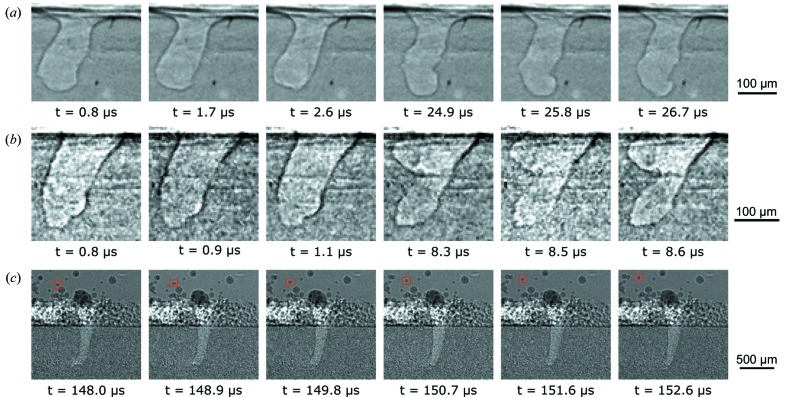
High-speed X-ray images of (*a*) and (*b*) the laser AM process for the Al–Si10–Mg plate, and (*c*) the powder-bed fusion process for Ti–6Al–4V. The frames are cropped such that the details of the vapor depression can be clearly identified as *e.g.* oscillations in the liquid–vapor surface. The experimental parameters were: (*a*) laser power 520 W, scan speed 0.8 m s^−1^, frame rate 1.08 MHz and exposure time 200 ns; (*b*) laser power 468 W, scan speed 0.6 m s^−1^, frame rate 10 MHz and exposure time 50 ns; (*c*) laser power 416 W, scan speed 0.7 m s^−1^, frame rate 1.08 MHz and exposure time 200 ns. The thickness of the plates was approximately 500 µm. The powder size for panel (*c*) was 15–45 µm.
